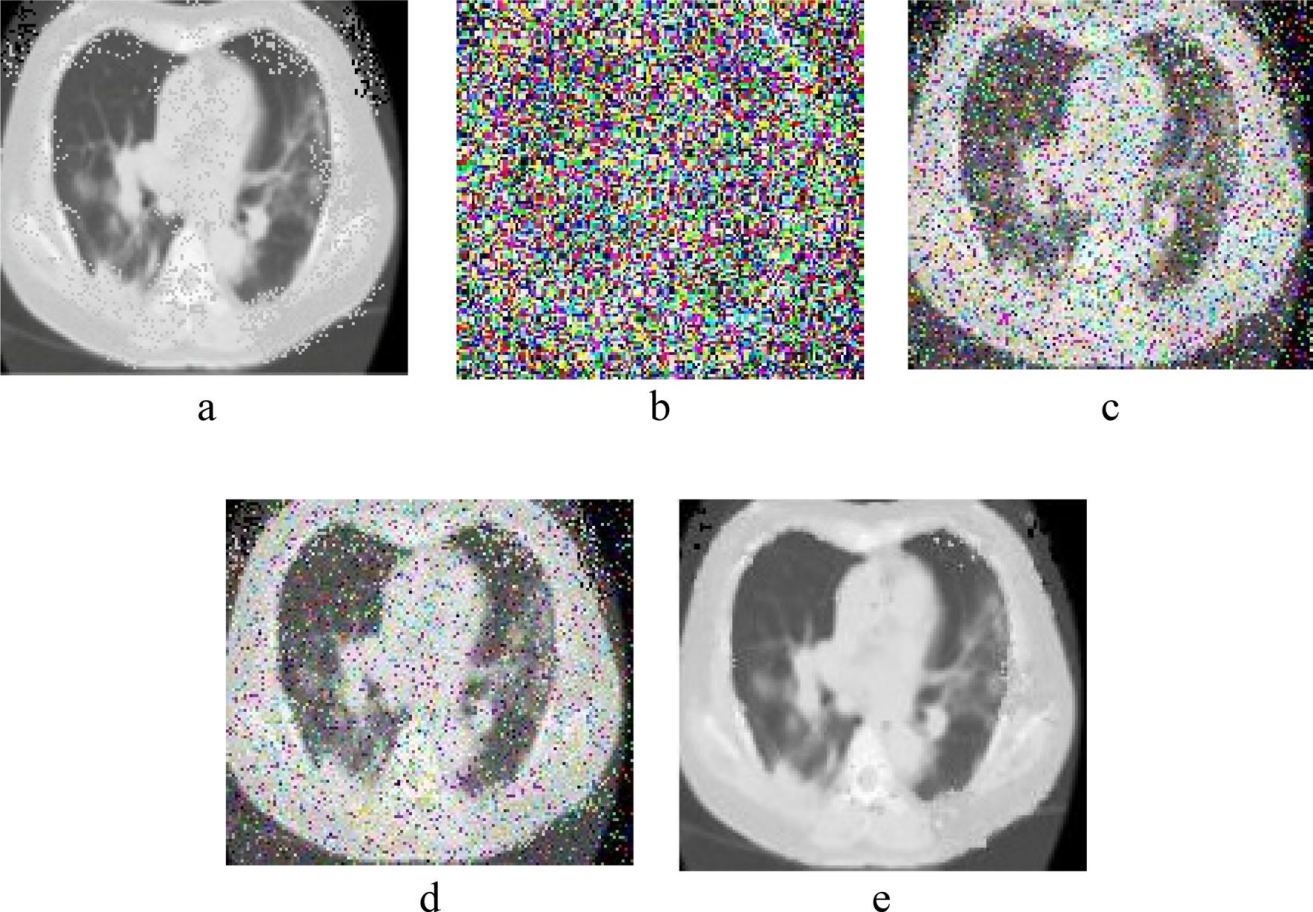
shows the normal, noisy and de-noised CT images. (a) Normal CT image having natural Impulse noise (b) Impulse noise corrupted image with 80% noise density (c) De-noised image with SM (d) De-noised image with BDND (e) De-noised image with proposed method.
